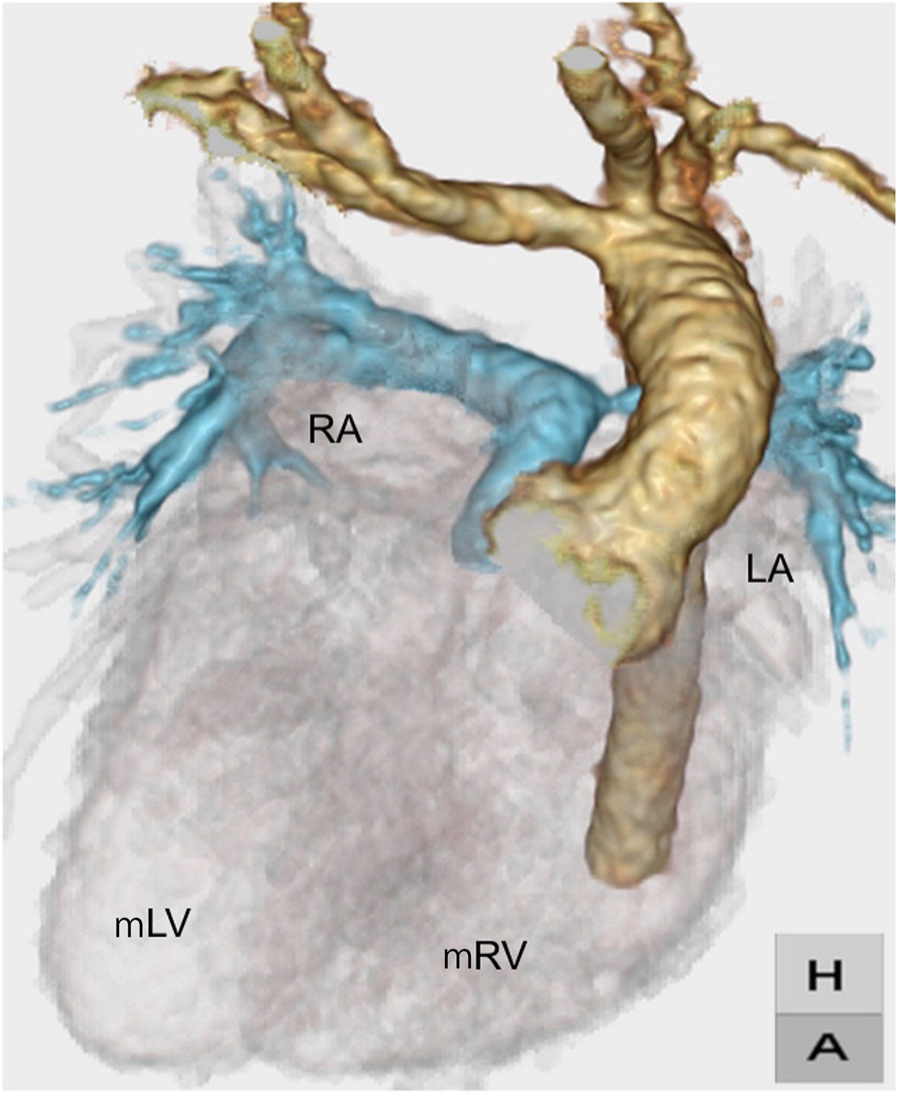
Three-dimensional computed tomography before initial palliation. LA, left atrium; mRV, morphological right ventricle; mLV, morphological left ventricle
